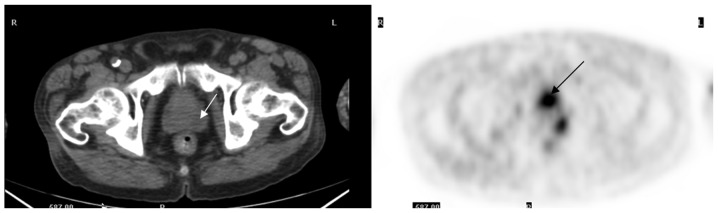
Transaxial fluorine-18 fluorodeoxyglucose (FDG) positron emission tomography (PET)-computed tomography (CT) images obtained from a 72-year-old male (patient 21) following external beam radiotherapy for prostate cancer. The patient’s series prostate-specific antigen (PSA) levels were unremarkable until two years later when the PSA level was 7.8 ng/ml. FDG PET-CT demonstrated an FDG-avid lesion in the left-side of the prostate (indicated by the arrows), which was confirmed as recurrent adenocarcinoma by transrectal biopsy.
